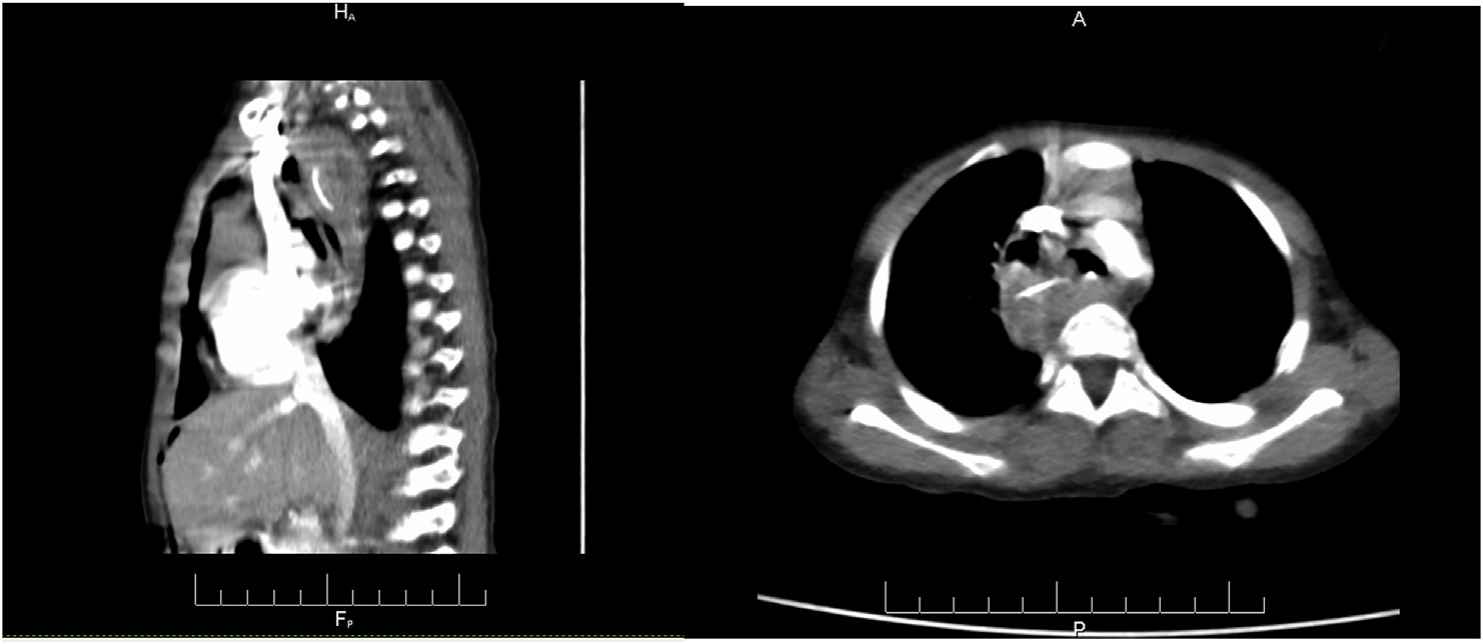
Enhanced chest CT scan axial and sagittal images show a linear dense object (arrow) in the middle mediastinum on the right side with surrounding soft tissue inflammatory phlegmon and multiple pockets of collections, causing narrowing the midesophagus.
